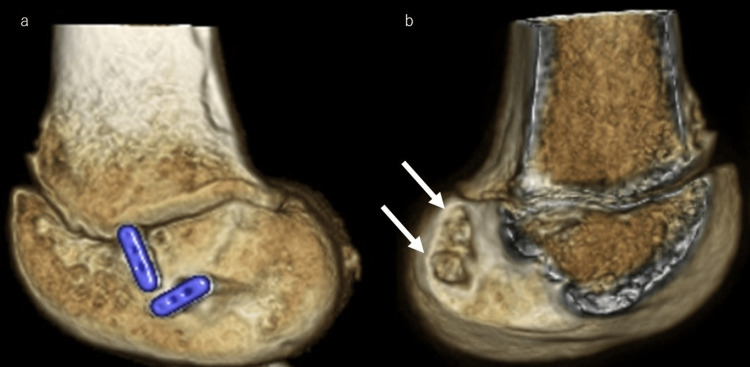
Three-dimensional CT after reconstruction of the left knee Femoral tunnels (A, B) are created within the epiphysis. White arrows indicate the locations of the femoral tunnel apertures.
